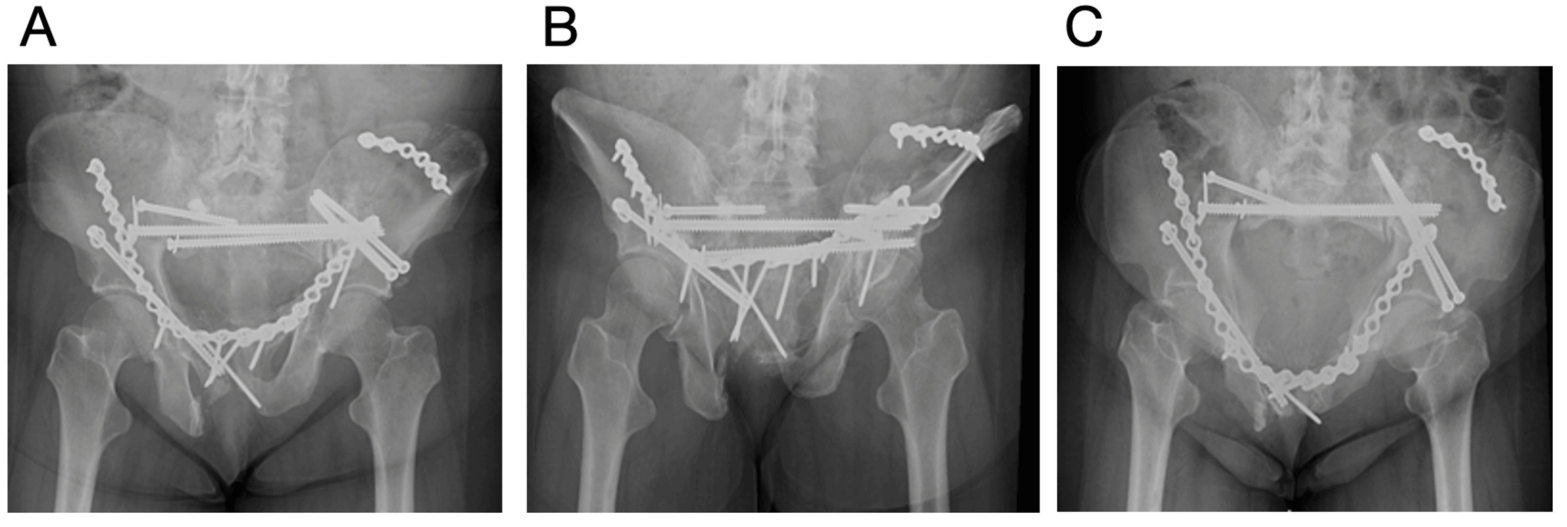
Radiograph status post re-revision surgery Images A (AP pelvis), B (pelvic outlet), and C (pelvic inlet) show the partial removal of the anterior plate with a new 16-hole recon plate overlapping the retained previous anterior plate. The previously placed right anterior column screw was removed and exchanged for a cannulated screw with a washer. The left LC-2 screw was maintained, but an additional left LC-2 screw was placed.
